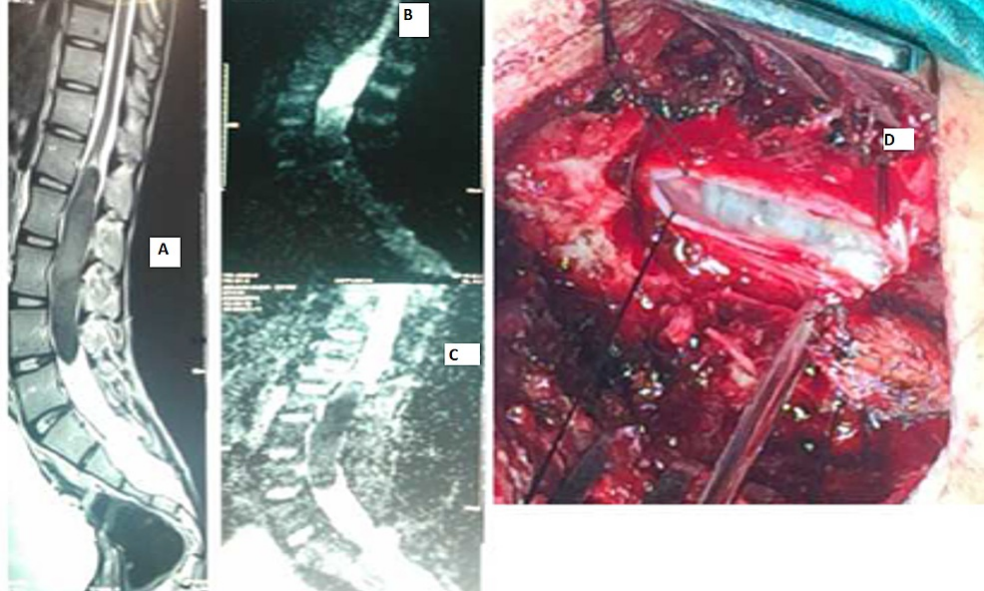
Lumbar epidermoid cyst A: MRI lumbosacral spine sagittal image with hypointense tumor mass on post contrast image. B: MRI lumbosacral spine with restriction on Apparent Diffusion Coefficient sequence. C: Diffusion-weighted image showing restriction. D: Intraoperative pearly white appearance of the cyst.
